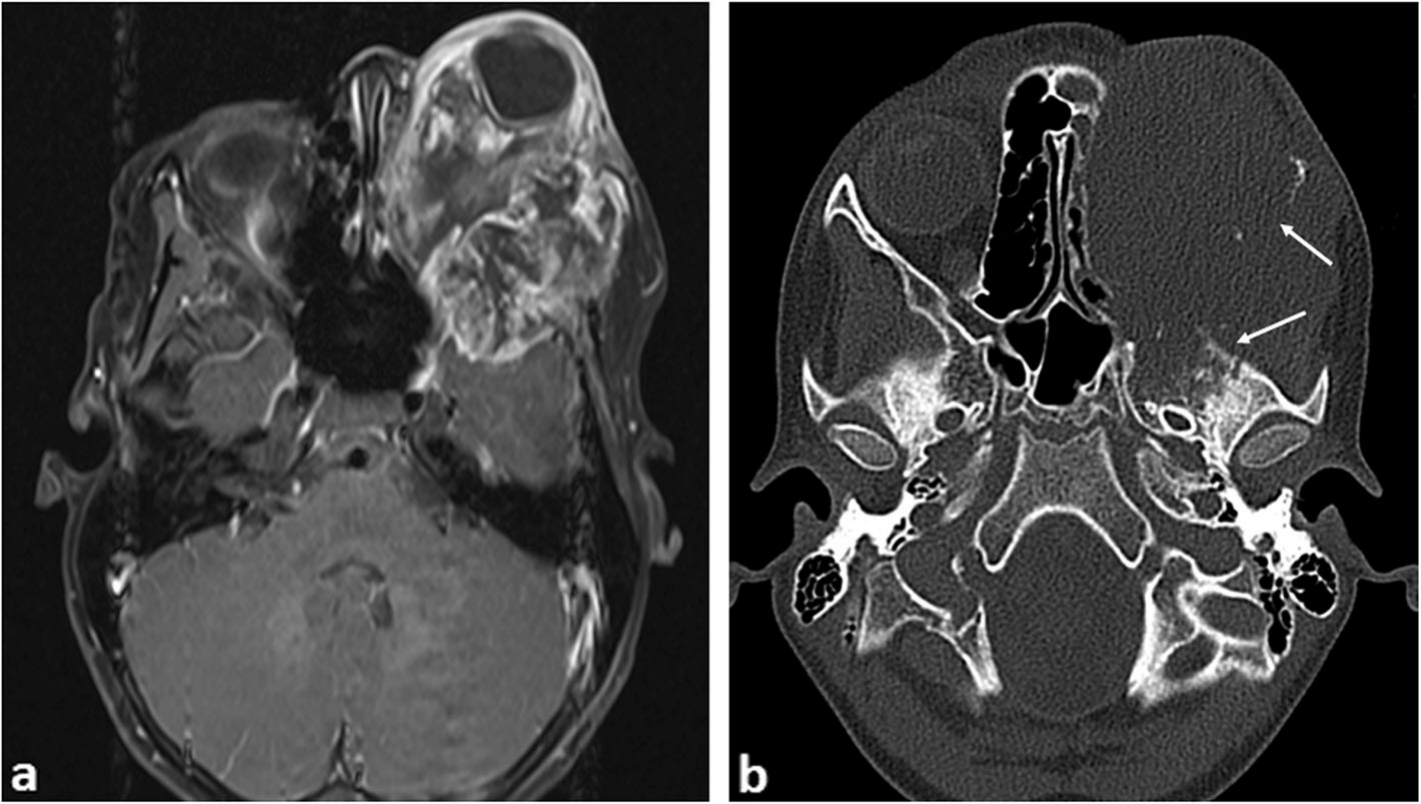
A 9-year-old boy with proptosis due to a left orbital rhabdomyosarcoma. a Axial post-contrast-enhanced T1-weighted MR image shows a large periorbital soft tissue mass with marked internal heterogenous enhancement invading the temporal fossa. b Axial CT image of the orbit demonstrates marked bony destruction of the skull and left ethmoid sinuses (arrows)
